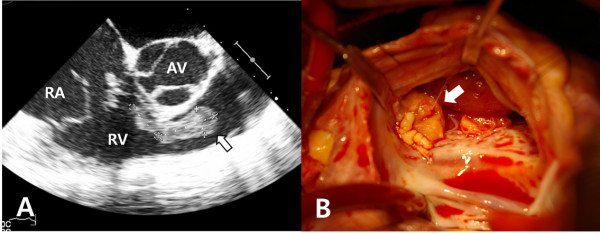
Preoperative transesophageal echocardiogram and gross operative findings. A. The tricuspid valve had prolapsed leaflets with coaptation failure and a mobile mass of 2.4 cm × 0.8 cm (white arrow) attached to the ventricular septum under the valve. B. An abscess lump (white arrow) was attached to ventricular septum; it was just at the base of the disrupted medial papillary muscle. (AV = aortic valve; RA = right atrium; RV = right ventricle).
